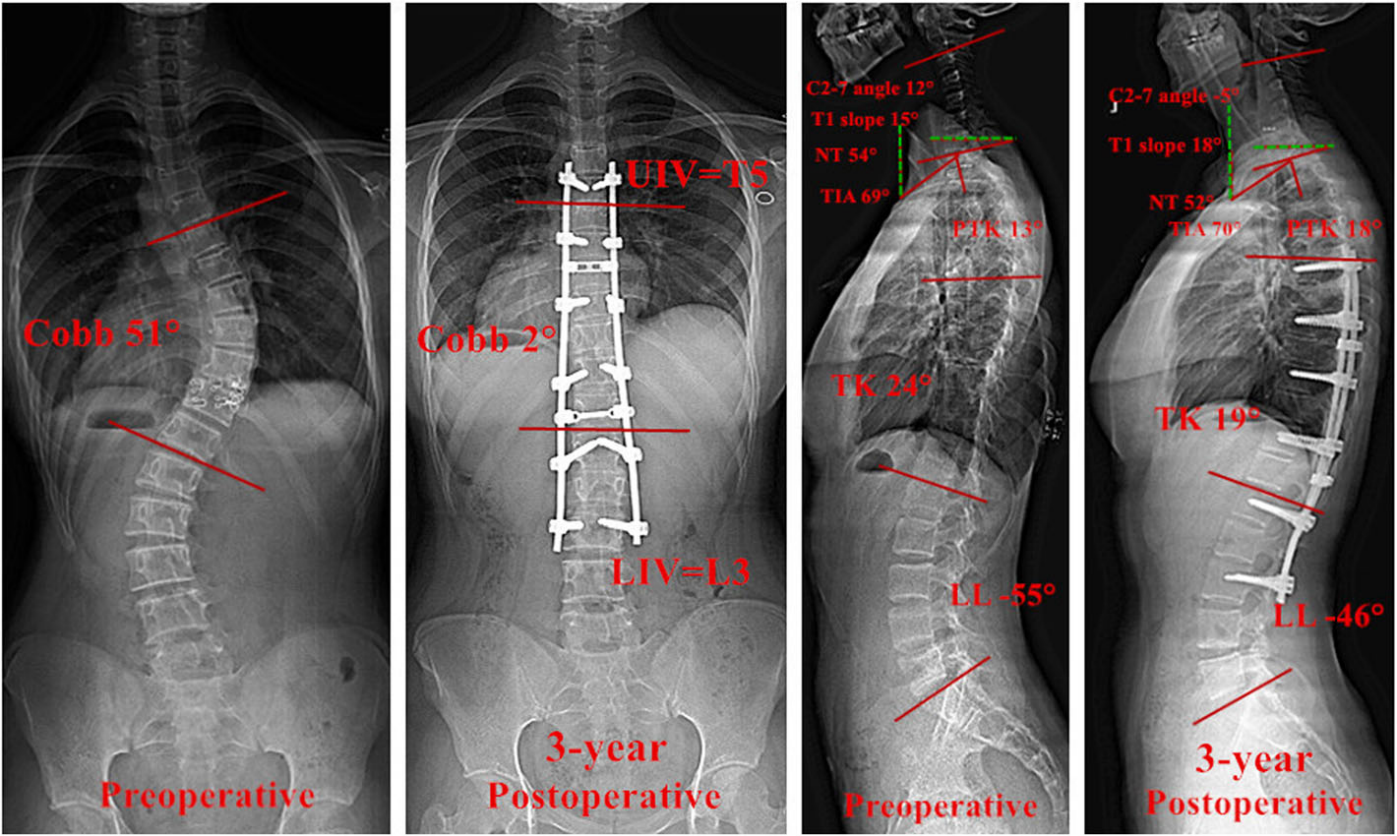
The pre- and 3-year postoperative radiographs of a Lenke 1 patient with AIS who had preoperative CK and postoperative CL. The coronal Cobb angle of the *main curve* was corrected from 51° preoperative to 2° postoperative (the rate of corrective Cobb angle was 96.1%). The C2-7 angle varied from 12° preoperative to −5° postoperative. The PTK increased from 13° preoperative to 18° postoperative. The TK decreased from 24° preoperative to 19° postoperative. AIS, adolescent idiopathic scoliosis; CK, cervical kyphosis; CL, cervical lordosis; PTK, proximal thoracic kyphosis; TK, thoracic kyphosis
